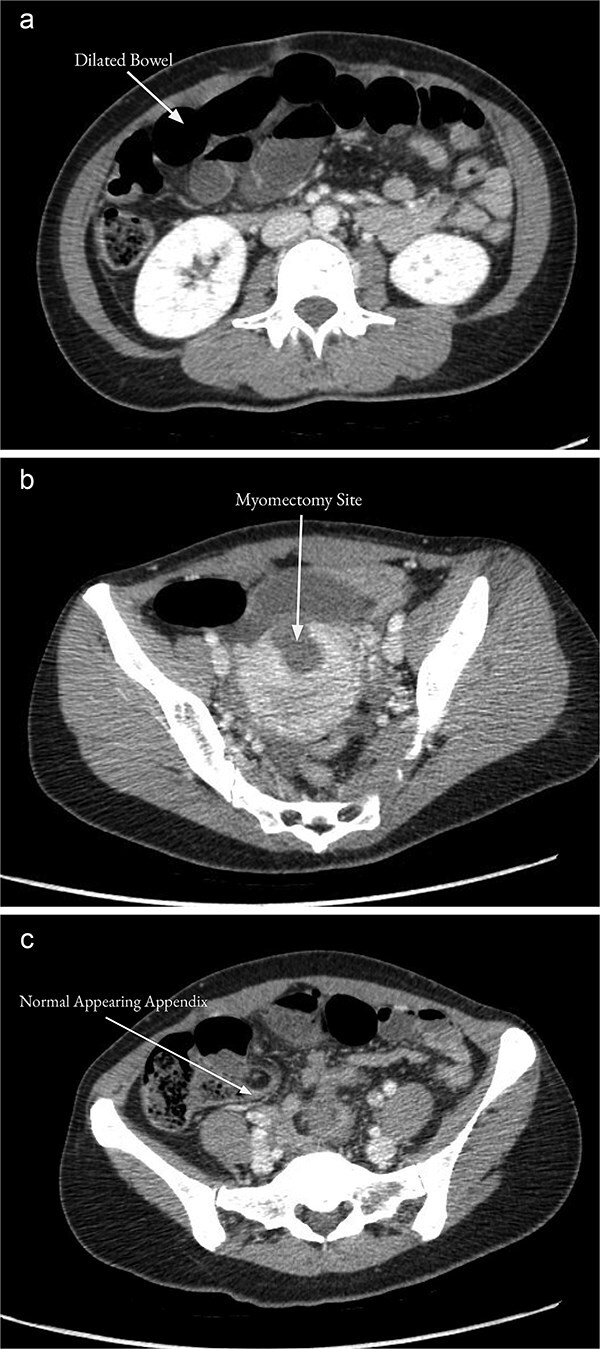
CT of the abdomen and pelvis with contrast on the day of admission showed multiple distended mid-distal small bowel loops indicating a possible small bowel obstruction (a). There was focal low density along the uterine myometrium consistent with the history of recent myomectomy (b). The appendix appeared normal (c).
